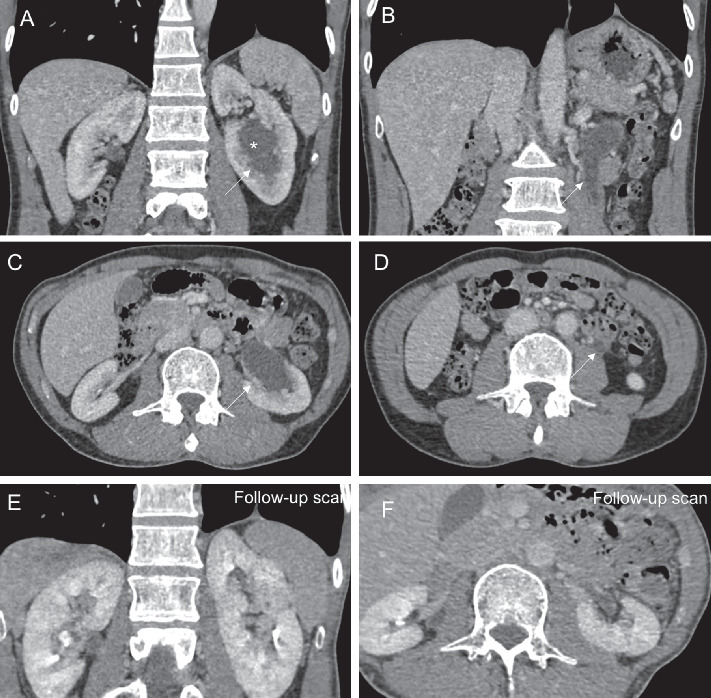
Selected contrast-enhanced CT images of the upper abdomen in coronal and axial planes showing initial (a–d) and posttreatment follow-up (e, f) scans. The images of the initial scan show moderate hydronephrosis and hydroureter (thin white arrows) of the left lower moiety system (^*∗*^), which are largely resolved in the follow-up scan. There were no calculi, ureteric mass, or abdominal lymphadenopathy.
